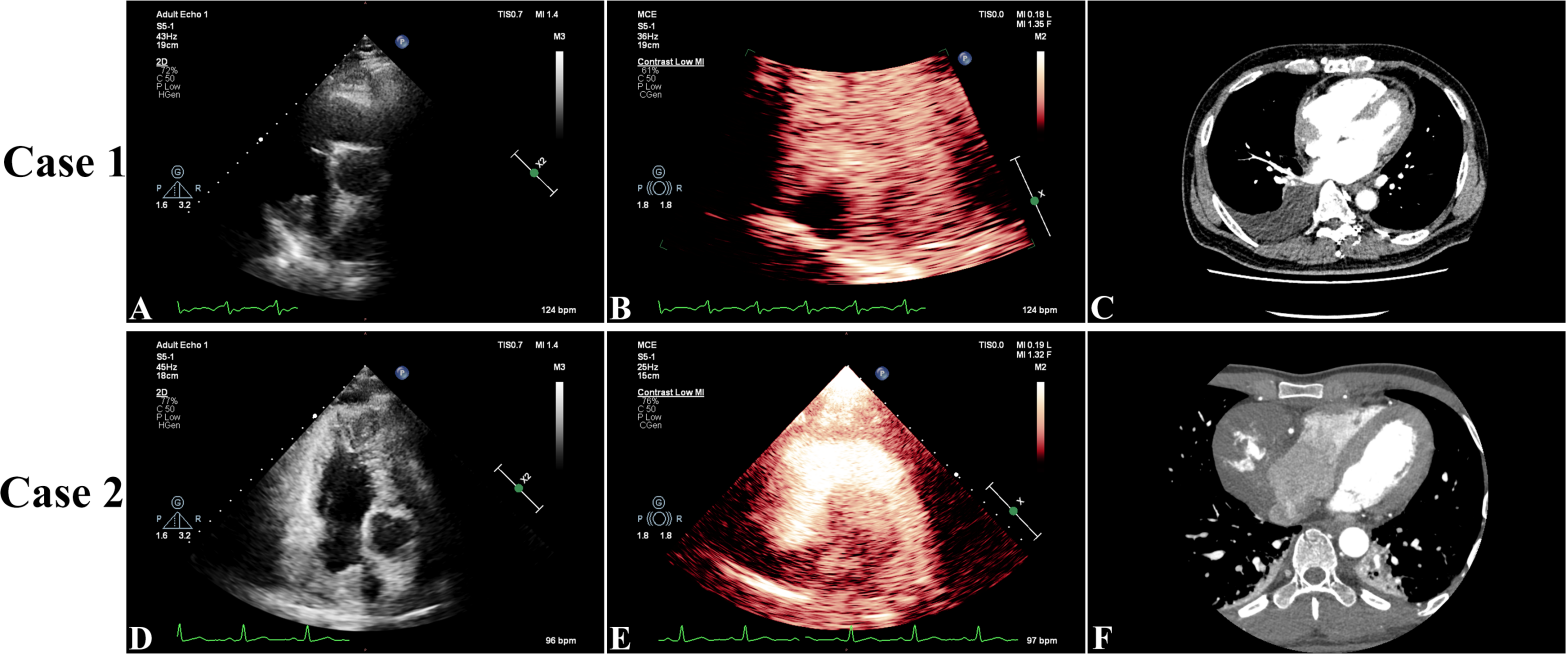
In Case 1, preoperative three-dimensional transthoracic echocardiography showed a hypoechoic mass in the right atrium (RA), pericardial thickening, and a left ventricular ejection fraction of 44% with reduced ventricular wall motion (A). Contrast-enhanced ultrasound showed a filling defect in the RA and no wash-in of the hypoechoic mass (B). Arterial phase computed tomography angiography showed that the mass (measuring 3.7 × 3.0 cm) was located on the right posterior wall of the RA and had a broad base (C). In Case 2, transthoracic echocardiography showed a mass with medium-to-low echogenicity, with a broad basal change and an unclear border with the posterior wall of the RA, that extended toward the opening of the superior vena cava. The LVEF was measured at 60% with normal wall movement (D). Contrast-enhanced ultrasound showed a filling defect in the RA with a moderately enhancing mass (E). Computed tomography angiography showed a hypodense right atrial mass (measuring 7.9 × 6.0 cm) with heterogeneous enhancement and pericardial thickening (F).
